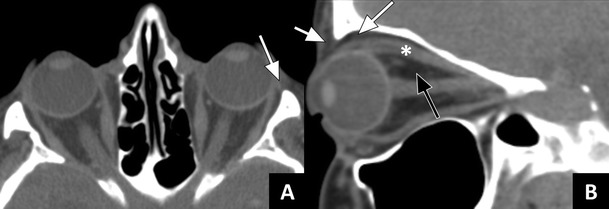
Orbital anatomy: a) Axial CT images in soft tissue window showing the orbital septum (arrow). b) Sagittal image showing the preseptal fat (short arrow), and the postseptal (extraconal) fat (long arrow); the intraconal fat (black arrow) is separated from the postseptal/orbital fat by the rectus muscles (* superior rectus muscle together with levator palpebrae superioris muscle)
